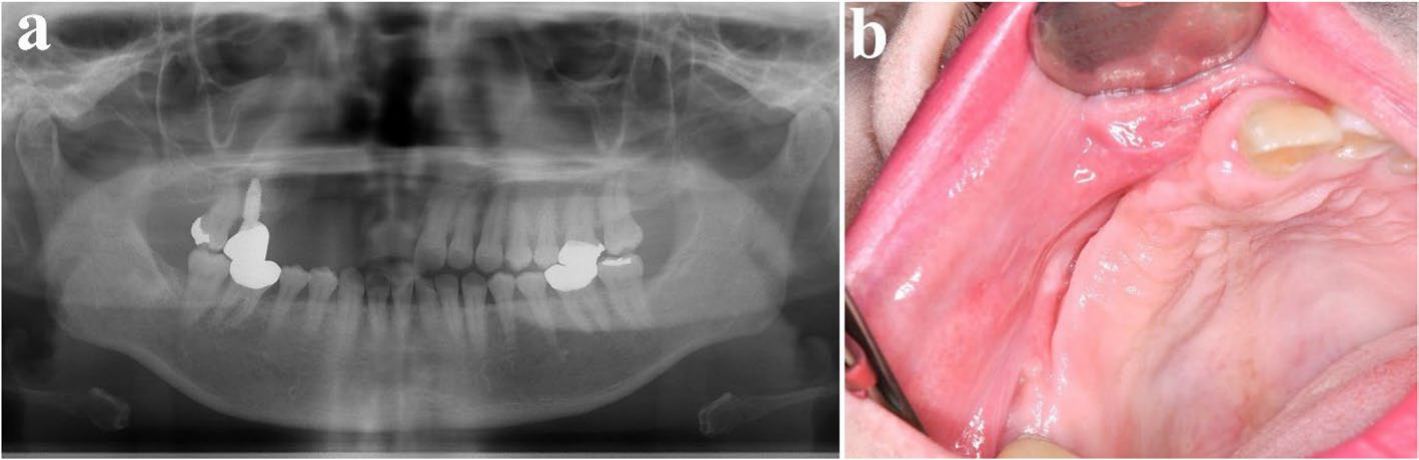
Postoperative radiograph and intraoral photograph a Panoramic radiograph at 2 years and 9 months postoperatively shows no evidence of recurrence. b Clinical photo at 2 years and 9 months
